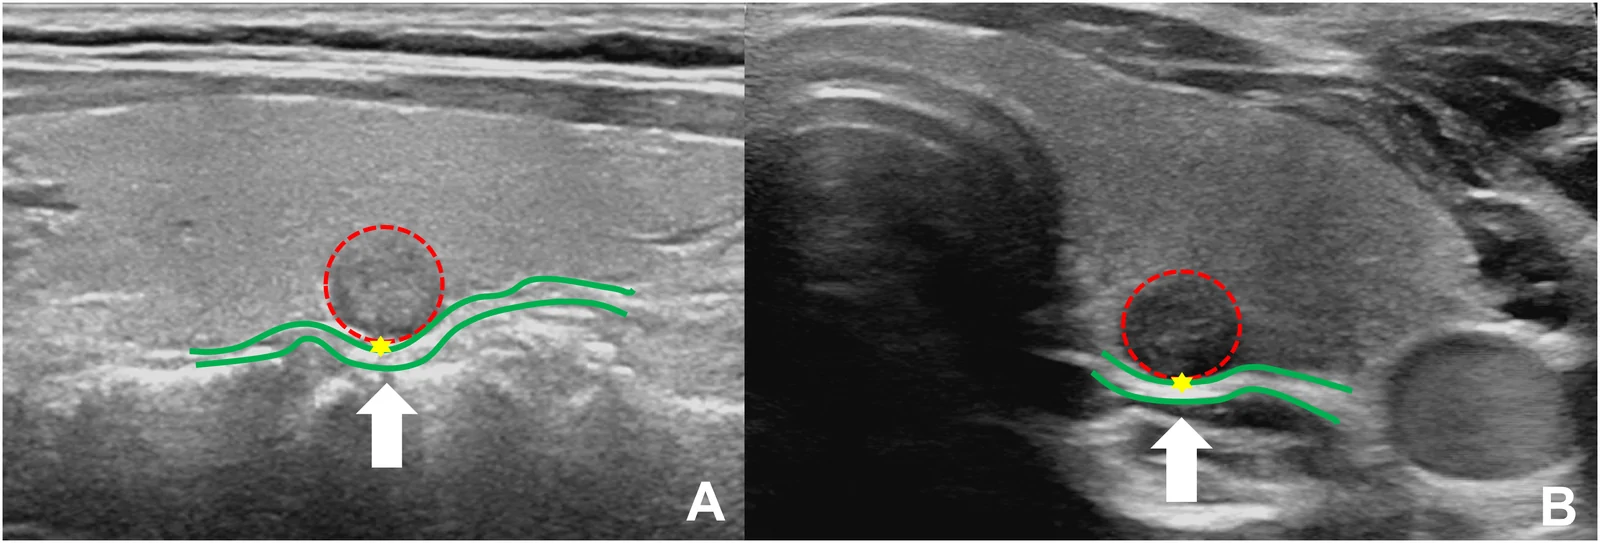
Preoperative ultrasound assessment of the spatial relationship between a thyroid nodule and the recurrent laryngeal nerve (RLN). (A) Longitudinal ultrasound view. (B) Transverse ultrasound view. In both images, the thyroid nodule is outlined by the red dashed circle, the white arrow indicates the RLN, the green lines delineate the course of the RLN, and the yellow star marks the tumor–RLN contact interface. Original images acquired at our institution.
